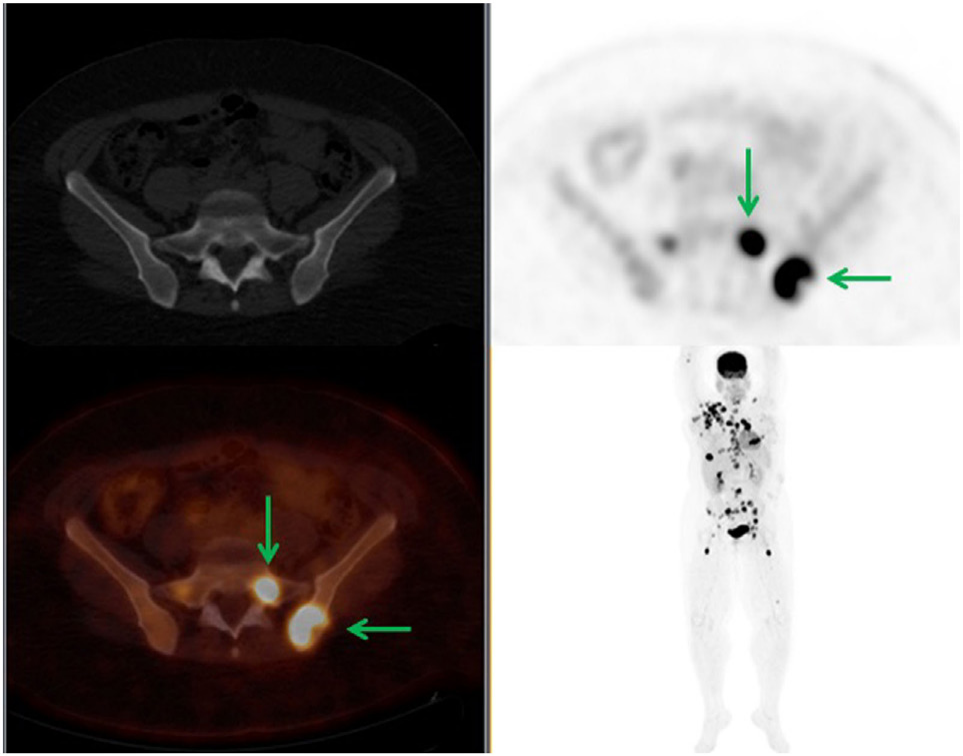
A 34-year-old female diagnosed with right breast cancer. 18F-FDG PET demonstrated widespread disease with PET-positive CT-negative SM. The FDG-avid left iliac bone and sacral lesions demonstrated no bone changes in the CT (green arrows). In this case, there was no change in staging; however, BMA was offered.
